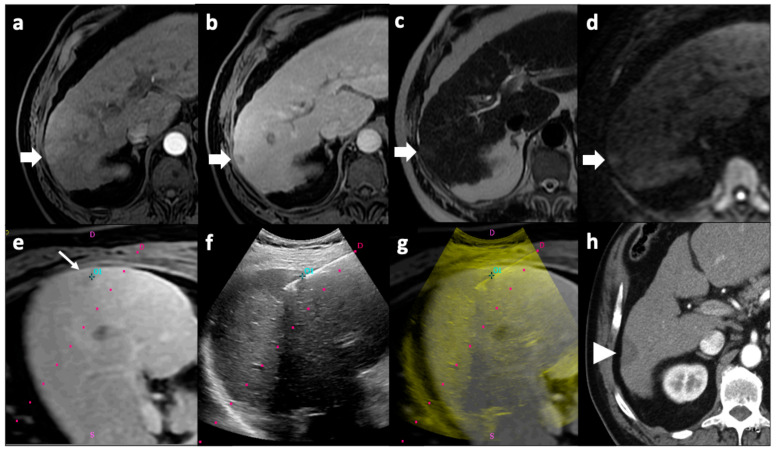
Microwave ablation of an HCC nodule. Pre-procedural MRI axial images demonstrated a focal right liver lesion with arterial wash-in (arrow, a), venous wash-out (arrow, b), hyperintensity on T2-weighted images (arrow, c), and diffusion restriction (arrow, d). The tumor was not clearly visible on US examination; thus, pre-procedural MR images in which the tumor was clearly detectable (thin arrow, e) were fused with real-time US images in which the microwave antenna could be monitored (f) during procedure; 01 corresponds to the point where the target lesion was selected on pre-procedural images. A fused MR–US image was produced showing the antenna correctly positioned inside the nodule (g). The red dot line in images (e–g) indicate the predicted path of the antenna when a needle guidance system is used, but in this case guidance was used only to enter liver parenchyma, not for precisely inserting the antenna inside the nodule. Follow-up axial CT imaging performed 1 month after the procedure showed a hypodense image consisting with the ablation zone (arrowhead, h) with no enhancement in the arterial phase, consistent with the absence of residual disease. CT: computed tomography; HCC: hepatocellular carcinoma; MRI: magnetic resonance; US: ultrasound.
